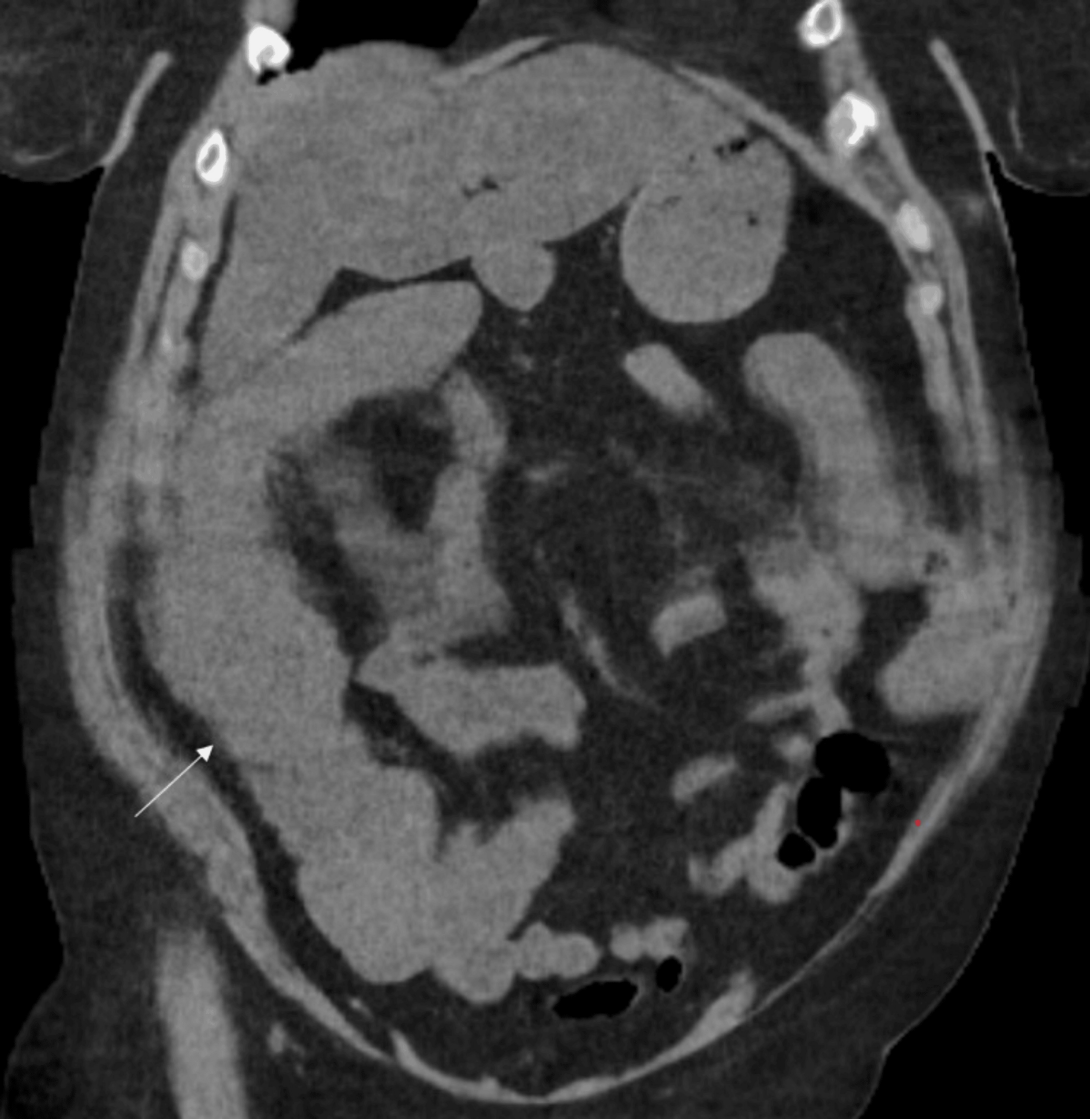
Representative coronal section of an abdominal CT scan showing fluid-filled large bowel loops (white arrow) CT: computed tomography
